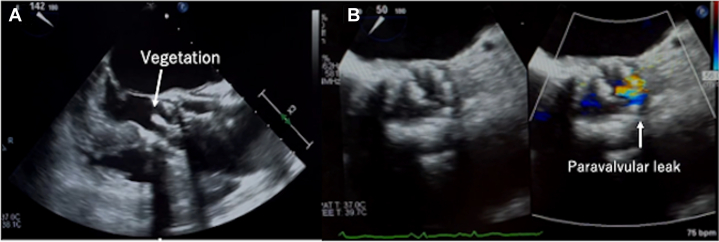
TEE of Aortic Valve Vegetation (A) TEE of aortic valve vegetation. (B) TEE with color Doppler of aortic valve paravalvular leak. TEE = transesophageal echocardiography.
